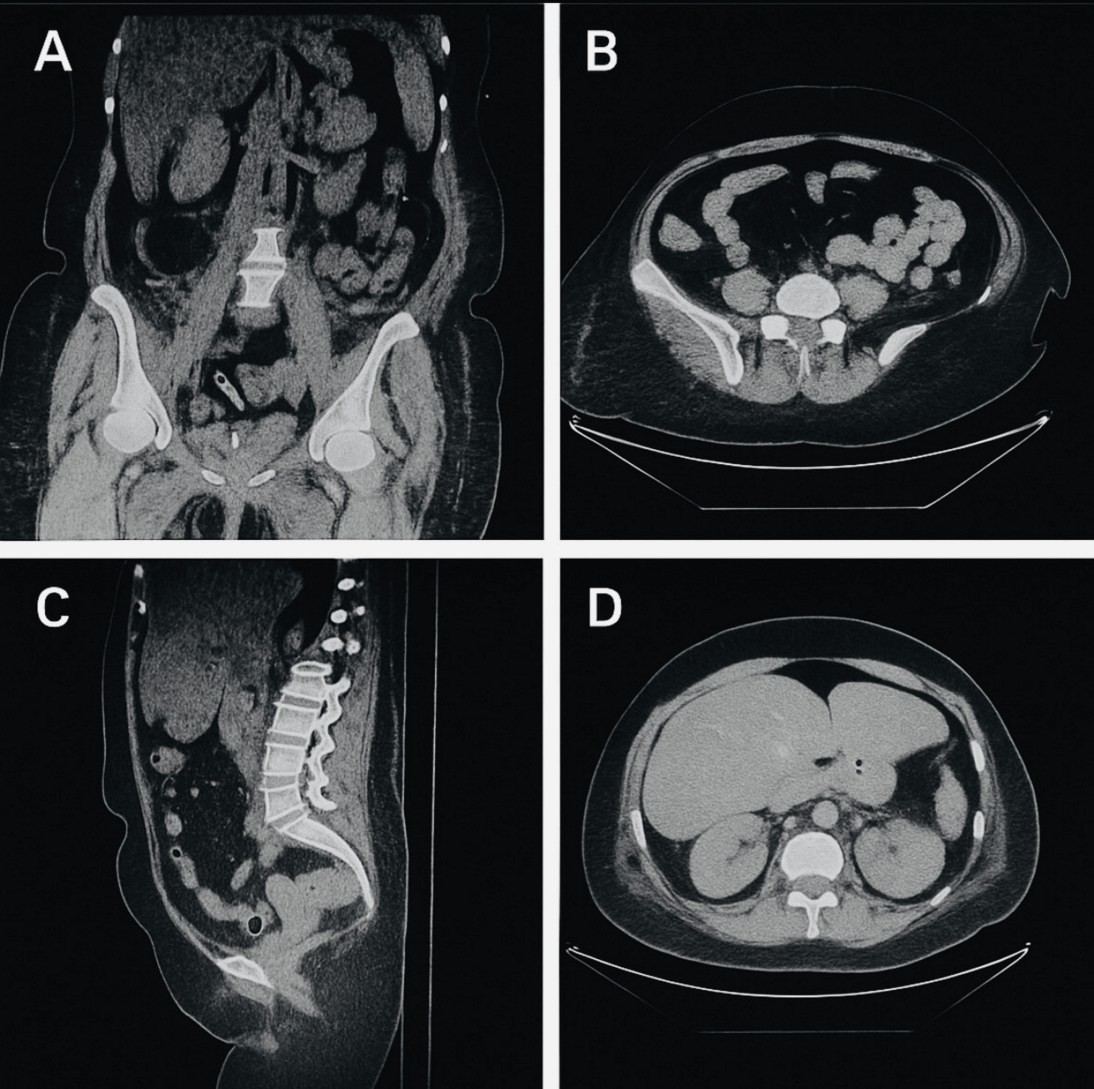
Contrast-enhanced CT images of the abdomen and pelvis A–D. Selected axial, coronal, and sagittal views. The scan showed diffuse hepatic steatosis, small bilateral pleural effusions with adjacent atelectatic/consolidative changes, and mild retroperitoneal fat stranding of uncertain significance. There was no hydronephrosis or abdominal collections. No significant lymphadenopathy or destructive bony lesions were identified.
